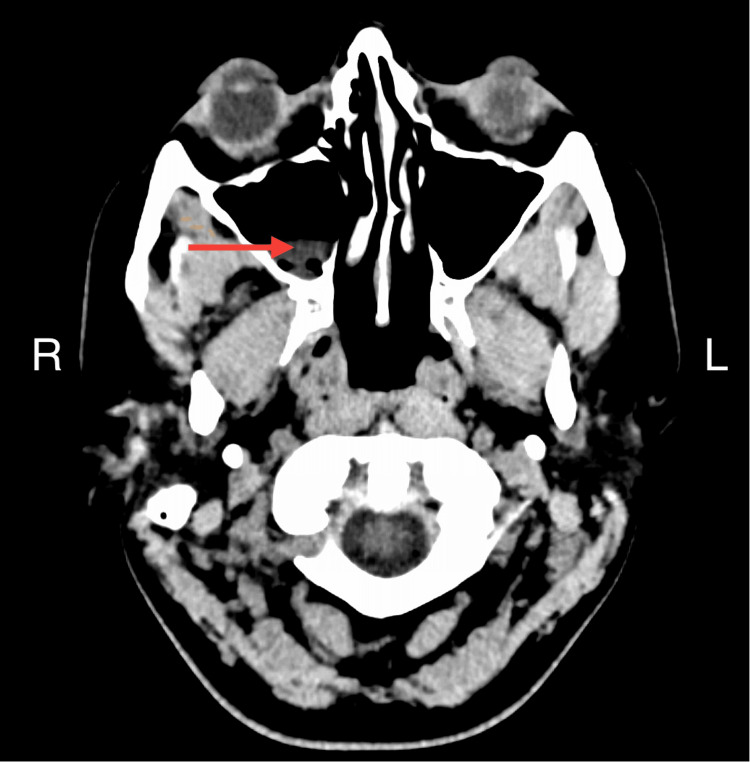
CT scan of the head The red arrow shows the right maxillary sinus with mucosal thickening suggesting sinusitis R: right, L: left
